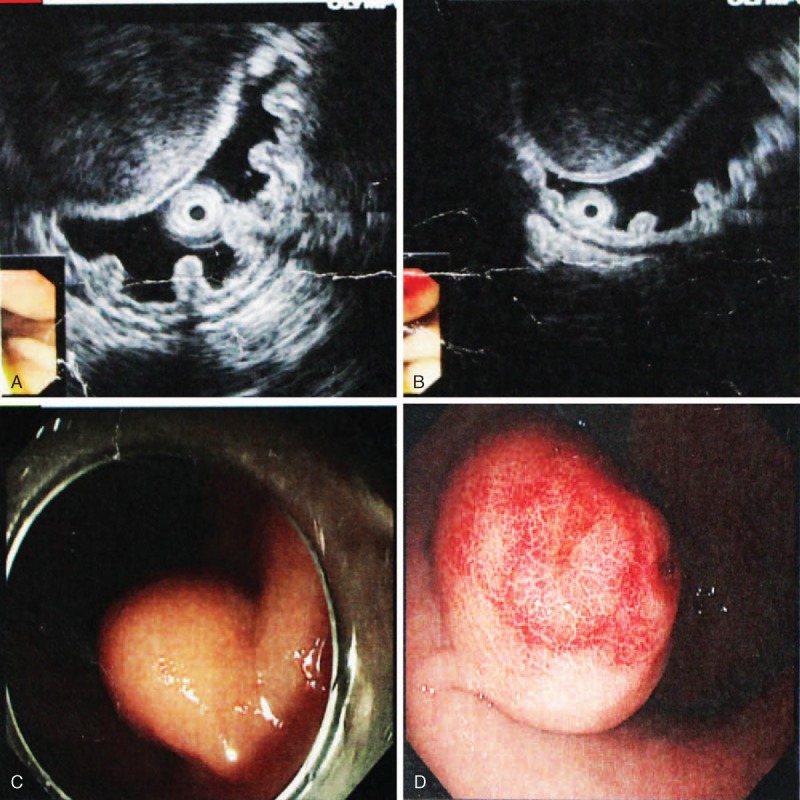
(A and B) Images of lesions located in the muscularis propria of the middle part of the stomach with a fairly clear boundary, as confirmed by EUS. (C and D) Endoscopic view of tumors located in the gastric body and tumors growing with an intragastric-type pattern. EUS = endoscopic ultrasonography.
